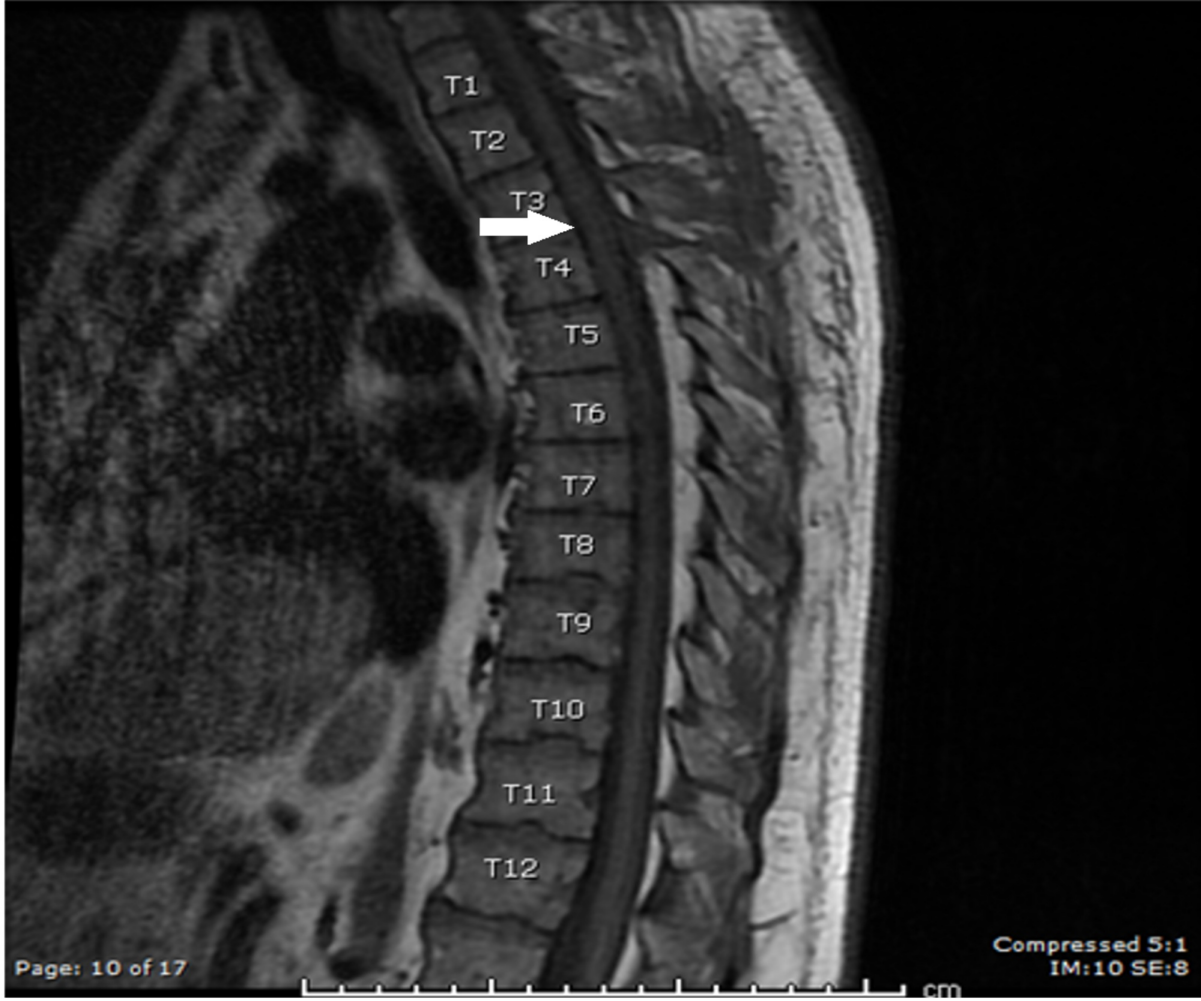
Postoperative T1 sagittal MRI with contrast MRI: magnetic resonance imaging
